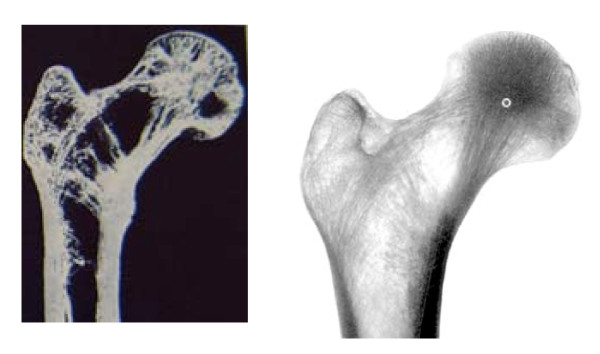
Anatomical bone slice of the proximal femur (85J old male) and x-ray in a.p. projection of the proximal femur
